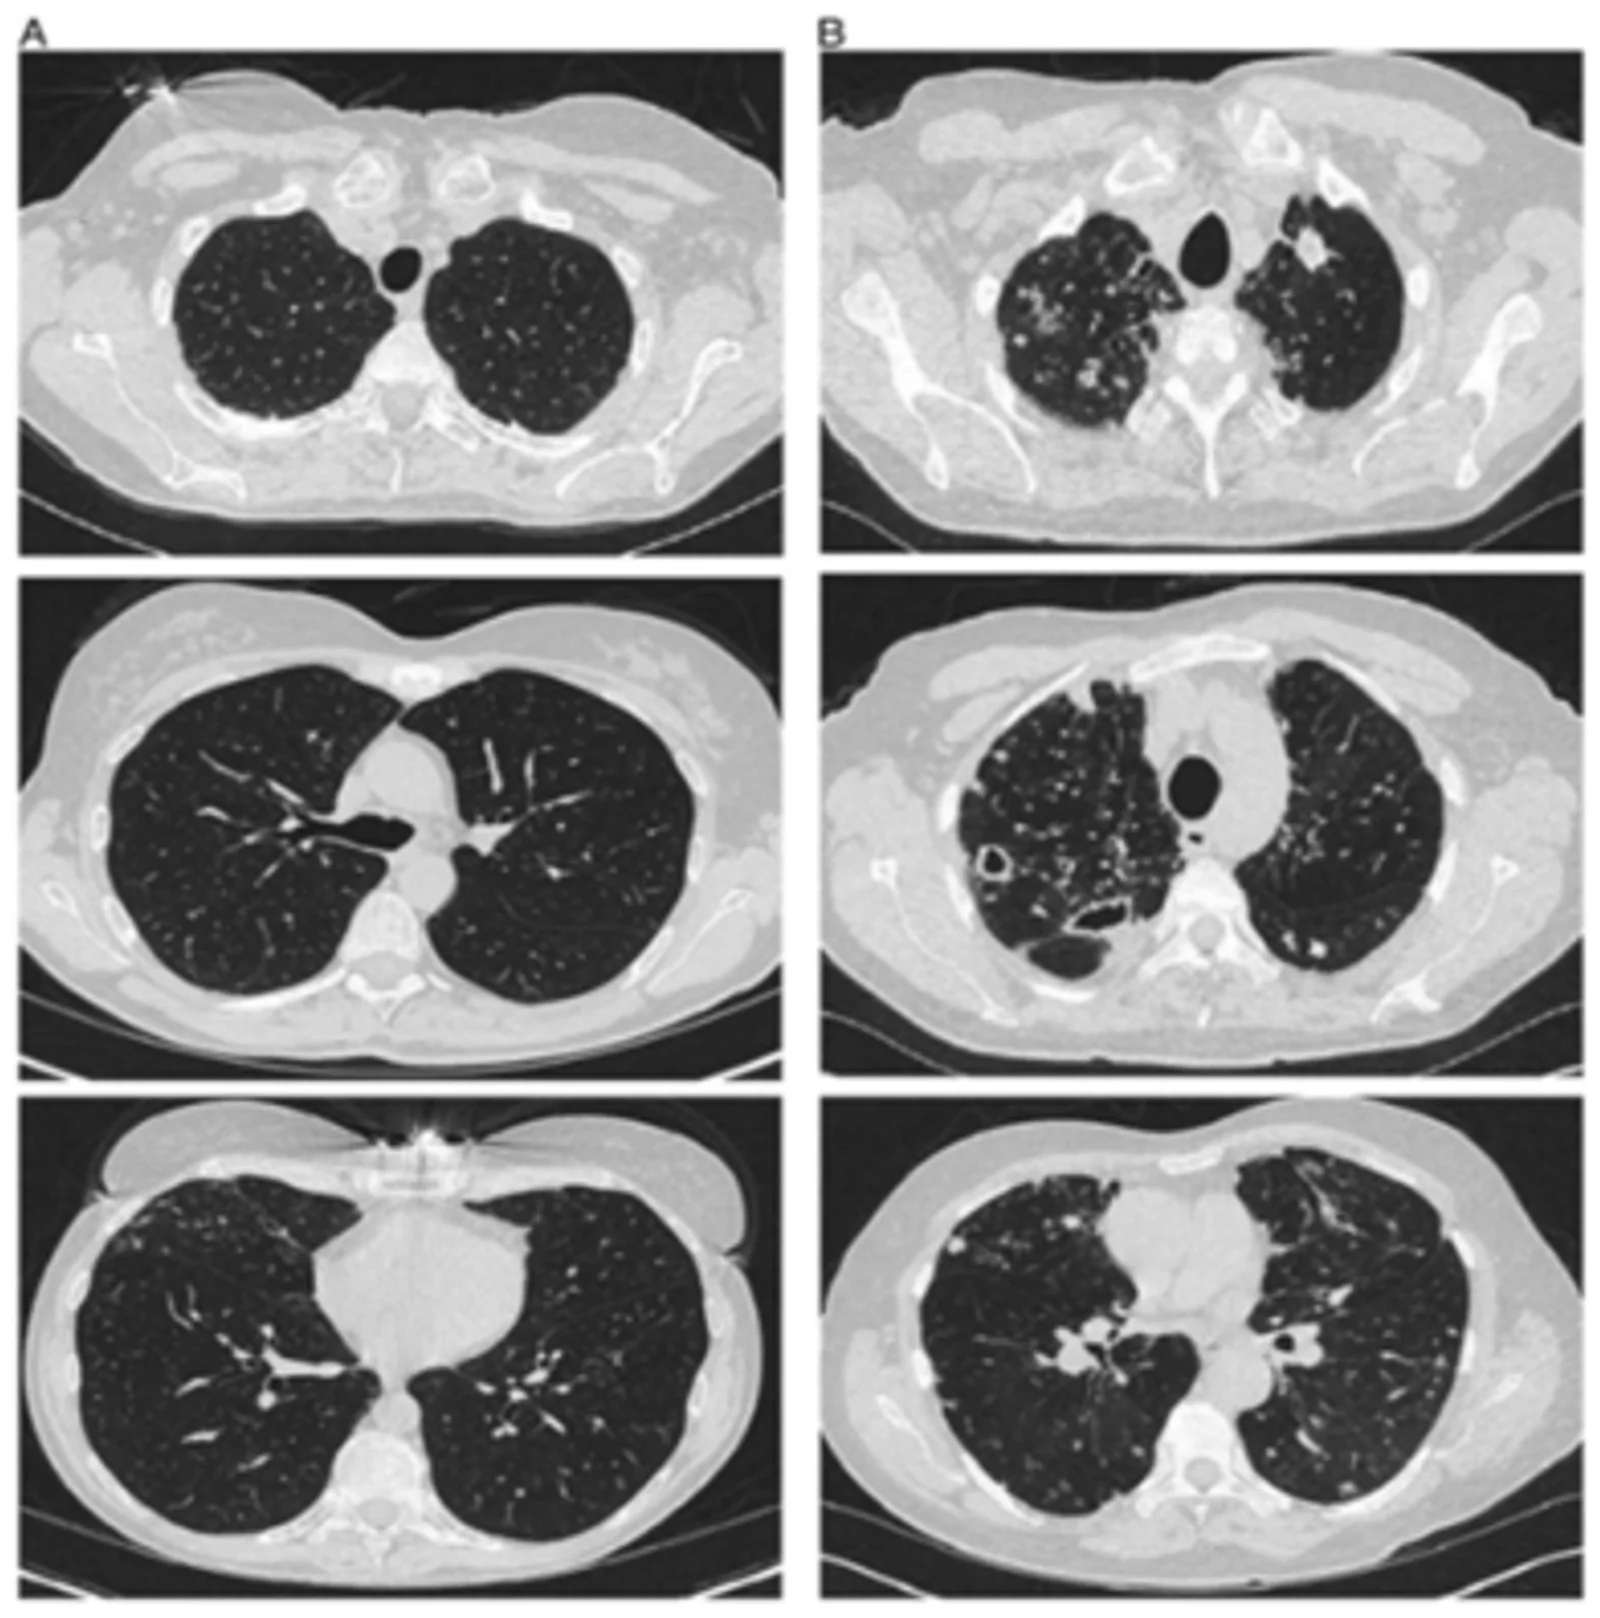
CT images showed: (A) Representative CT images of an NTM-C patient. Mild tubular bronchiectasis involving right middle and lower lobes and left lower lobe as well as nodular involvement of the corresponding lobes (Reiff score 3). (B) Representative CT images of an NTM-PD patient, characterized by 2 cavities in the right upper lobe and diffuse tubular bronchiectasis in all other lobes (Reiff score 8). There are nodules of varying sizes involving all lobes of lungs (37–39). CT, Computed Tomography; MDR-TB, Multi-drug resistant TB; NTM-C, Non-tuberculous mycobacteria colonization; NTM-PD, Non-tuberculous mycobacteria pulmonary diseases.
